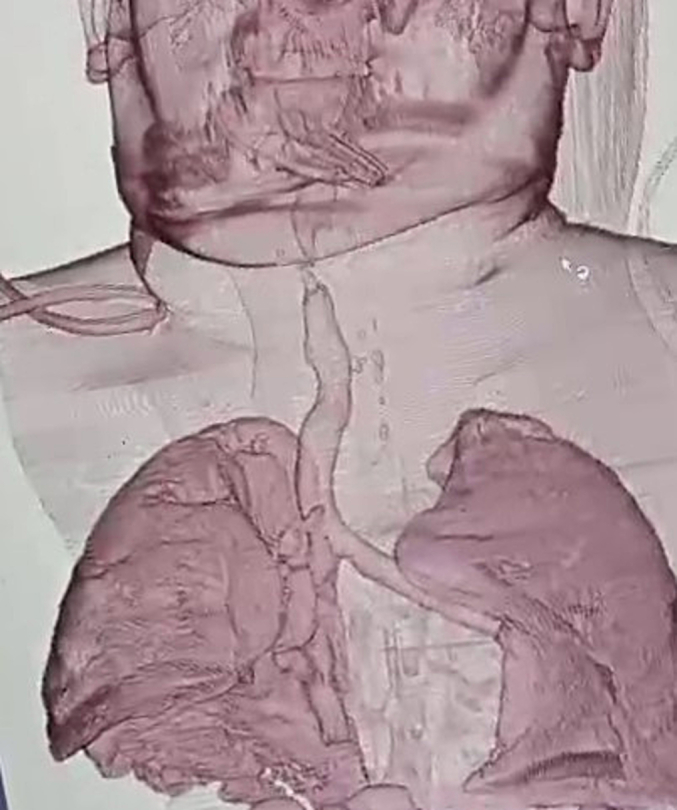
3D-reconstructed CT of the airway 2-years postop displaying no tracheal stenosis.
